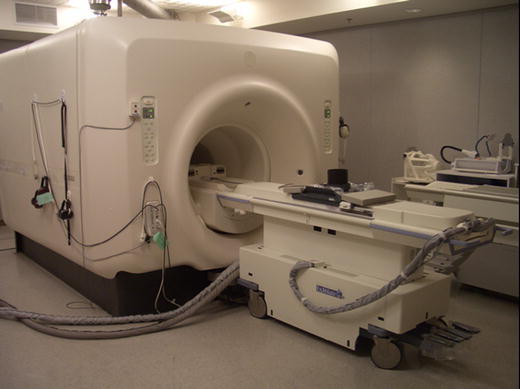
The ExAblate 2000™ FUS ablation device (InSightec, Ltd., Haifa, Israel) with a built-in 3-T MRI system [General Electric (GE) Medical Systems[ in the Lucas MR Imaging Centre, Stanford, CA
